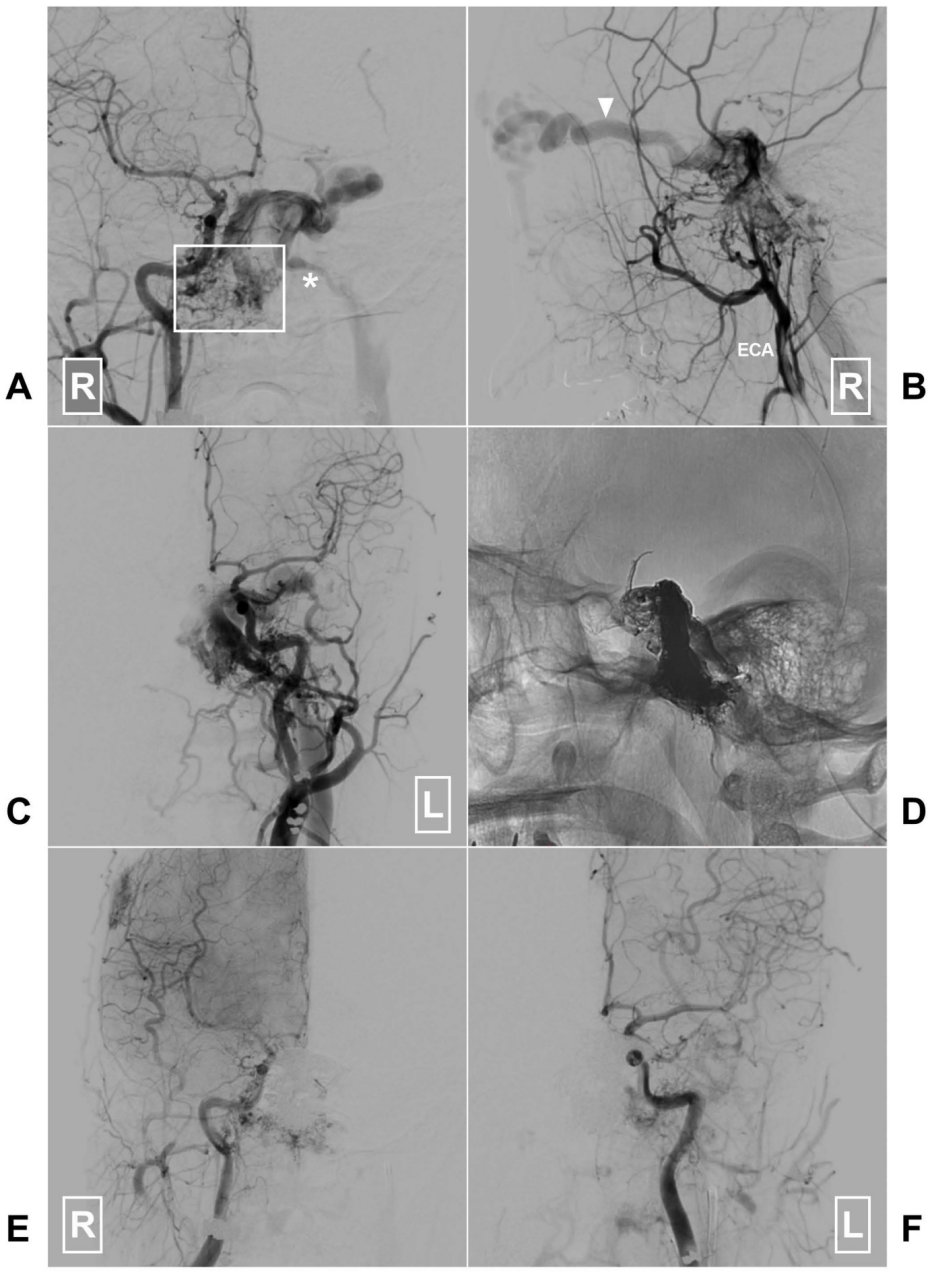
A, Angiogram of the right CCA in AP view reveals a clival DAVF (rectangle) draining to the contralateral IJV (asterisk) via the sigmoid sinus. B, Angiogram of the right ECA in lateral view shows there are multiple tiny feeding arteries. Arrow head denotes the ophthalmic vein drainage. C, Angiogram of the left CCA in AP view shows branches of the left ECA also supply the DAVF. D, X-ray of the cranium shows the Onyx casting after embolization of the through the left IJV. E-F, Angiogram of the right and left CCAs in AP view shows the DAVF is completely obliterated. Abbreviations: AP, anteroposterior; CCA, common carotid artery; DAVF, dural arteriovenous fistula; ECA, external carotid artery; IJV, internal jugular vein; L: left; R, right.
